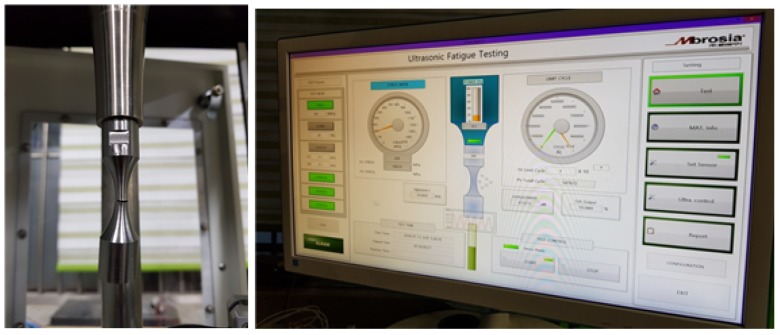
Ultrasonic fatigue test setup.
